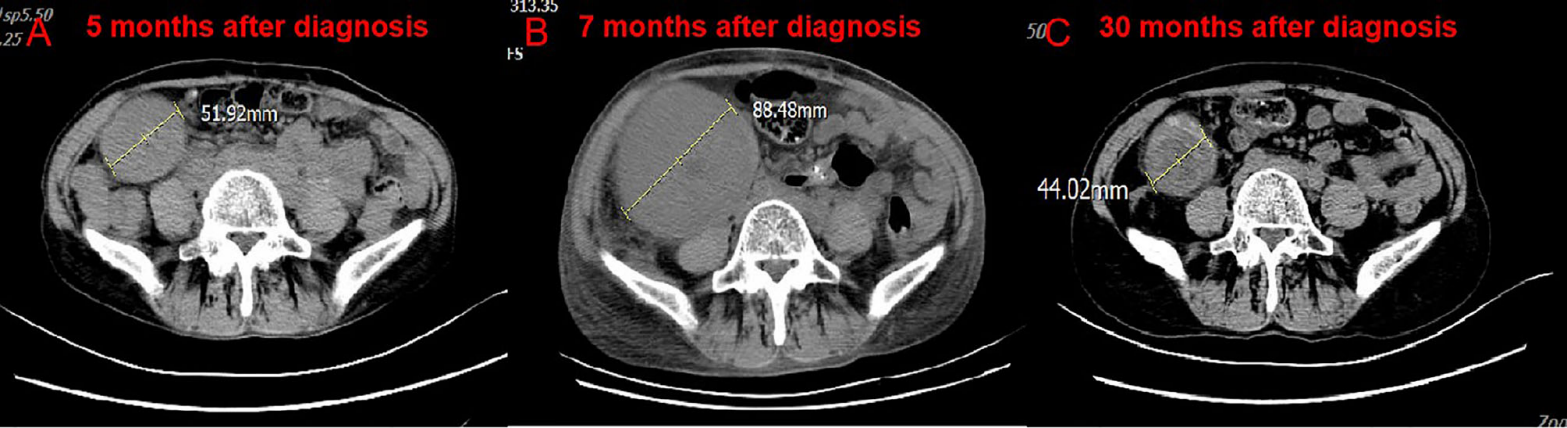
CT scan of the volume of transplanted kidney. Compare CT of April 18, 2019 (B) with February 20, 2019. (A) The volume of transplanted kidney increased after treatment of nivolumab. Compare CT of March 2, 2021 (C) with April 18, 2019. (B) The volume of transplanted kidney decreased after nivolumab stopped.
